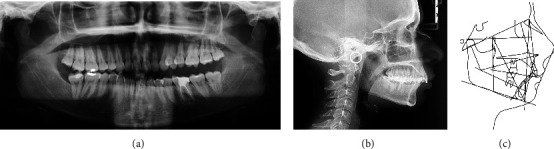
(a) Initial panoramic X-ray, (b) teleradiograph, and (c) cephalometry.
